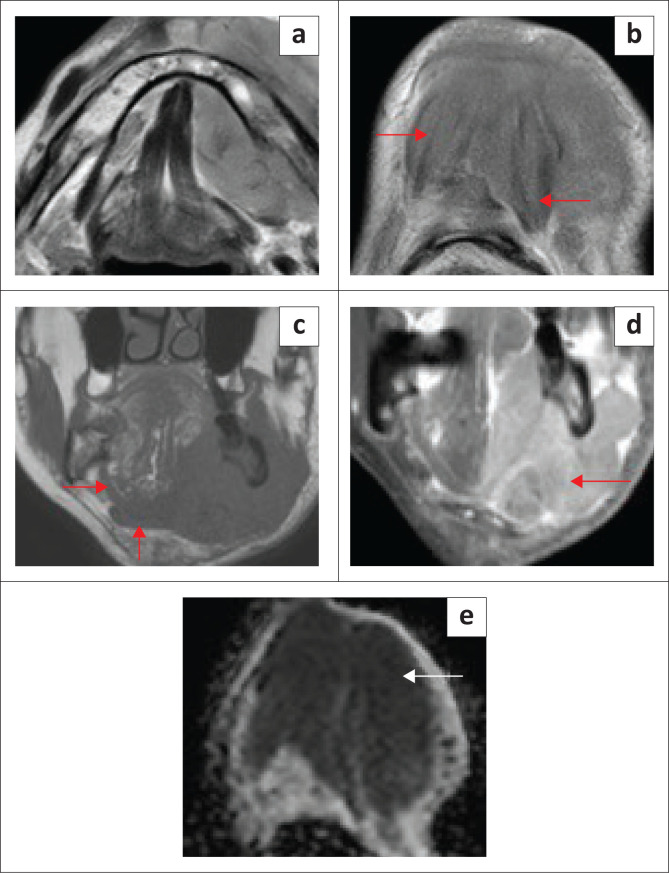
An 89-year-old male patient with aggressive diffuse large B-cell lymphoma. MRI neck; T2 axial (a, b), unenhanced T1 coronal (c) and post-contrast T1 fat sat coronal (d) demonstrate a large bulky soft tissue mass with full-thickness marrow infiltration and cortical destruction of the left mandibular body. The mass is involving the left floor of the mouth (FOM) and sublingual space with infiltration of the left muscles of the FOM, submental region and left submandibular gland. There is a marked restriction of diffusion on the apparent diffusion coefficient (ADC) map (e). T1 coronal (c) shows normal right mylohyoid and anterior belly of digastric muscles (red arrows).
